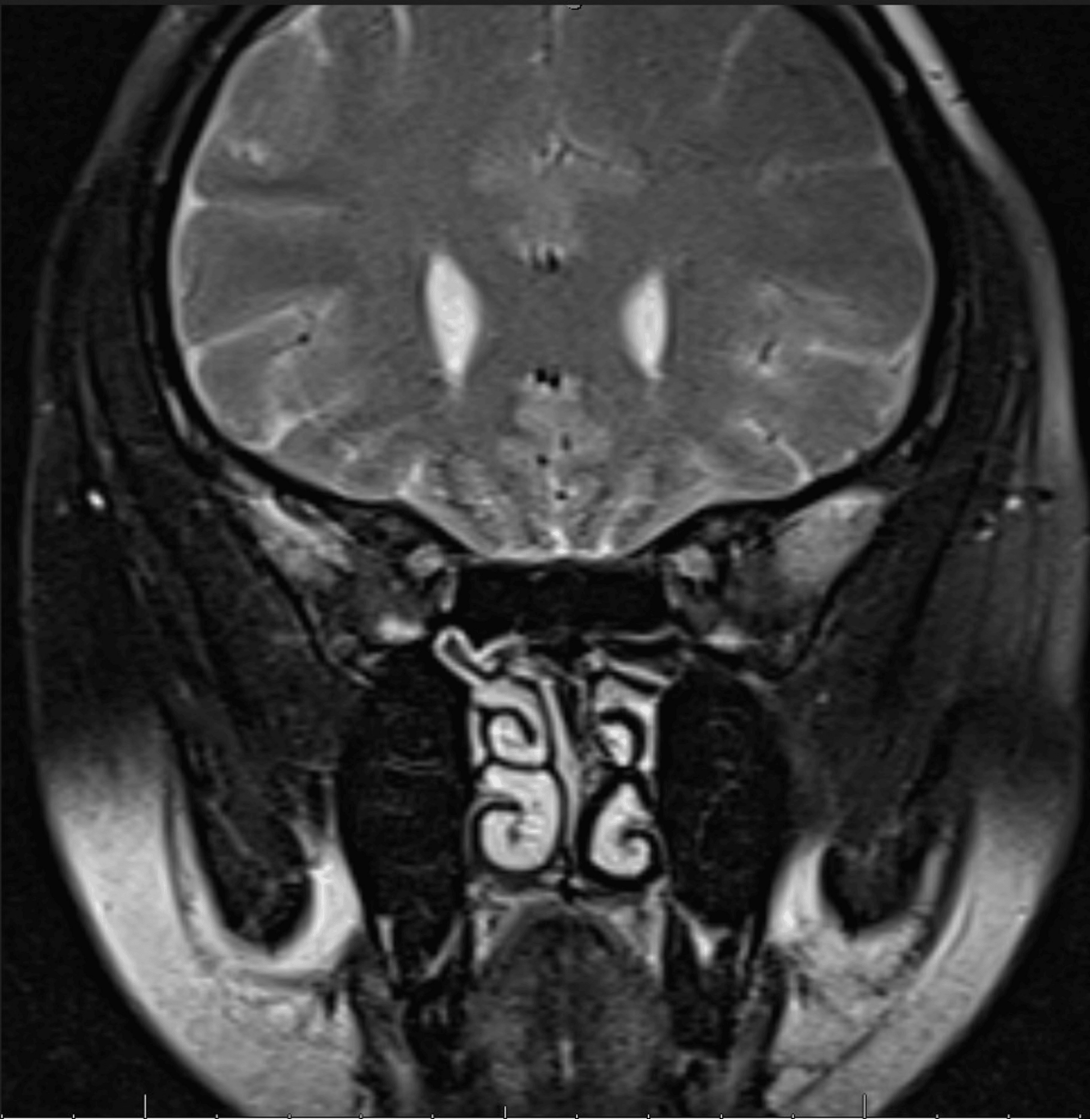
Coronal view of brain MRI showing normal pituitary gland morphology MRI: magnetic resonance imaging
